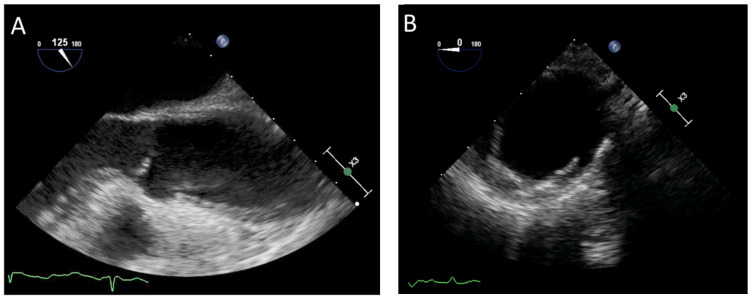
Transesophageal echocardiography assessment in two different patients with acute aortic syndrome: (A) intramural hematoma and (B) penetrating aortic ulcer.
